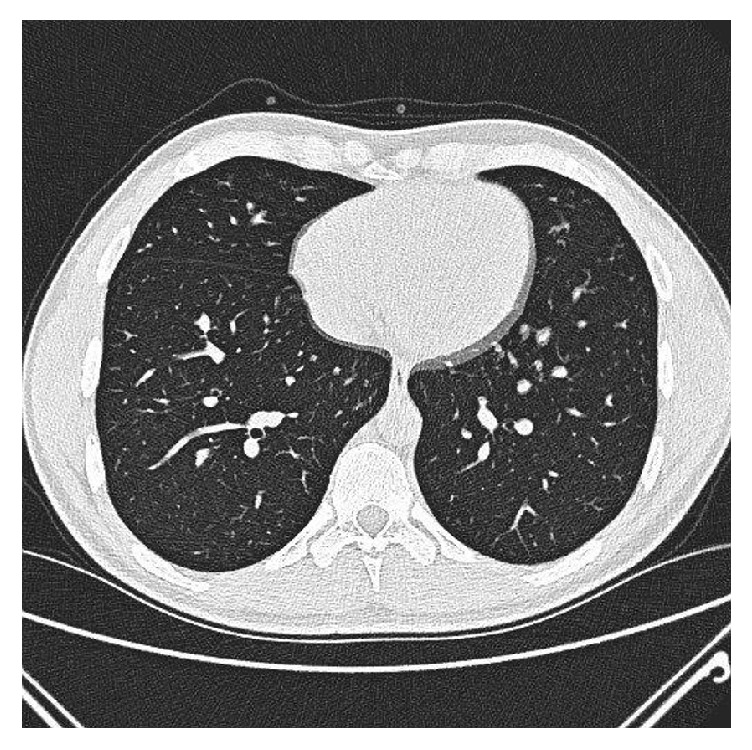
A normal chest CT scan of the patient obtained three years after the trauma.
